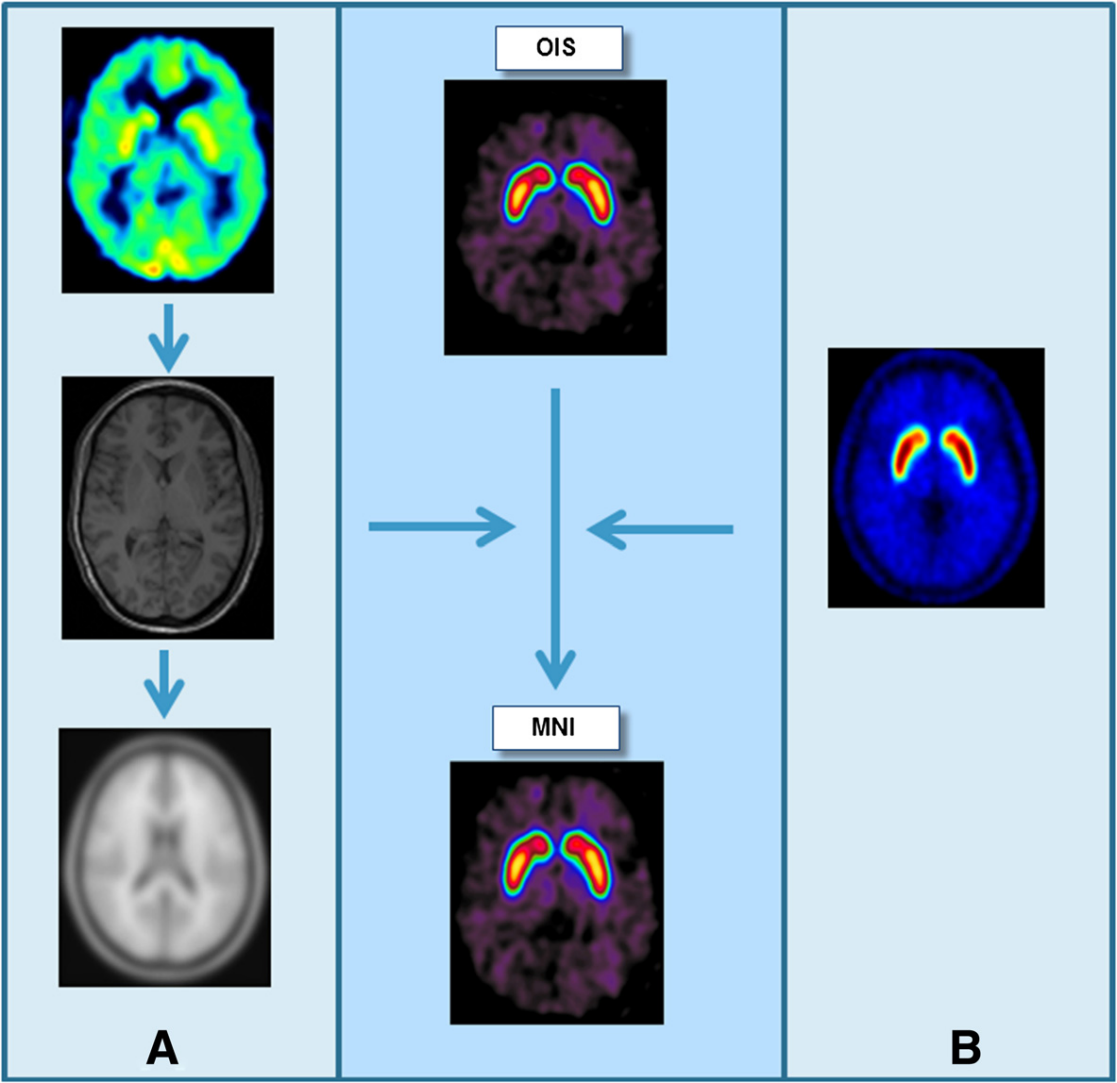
Normalization of the binding PET images. (A) Rigid co-registration of perfusion-like PET images to MRI (transformation matrix t1). Spatial normalization of MR images in original image space (OIS) into MNI space (t2). Combination of t1 and t2 to normalize the binding PET images into the MNI space. (B) Direct transformation of binding PET images to MNI space by normalization to the raclopride PET template.
